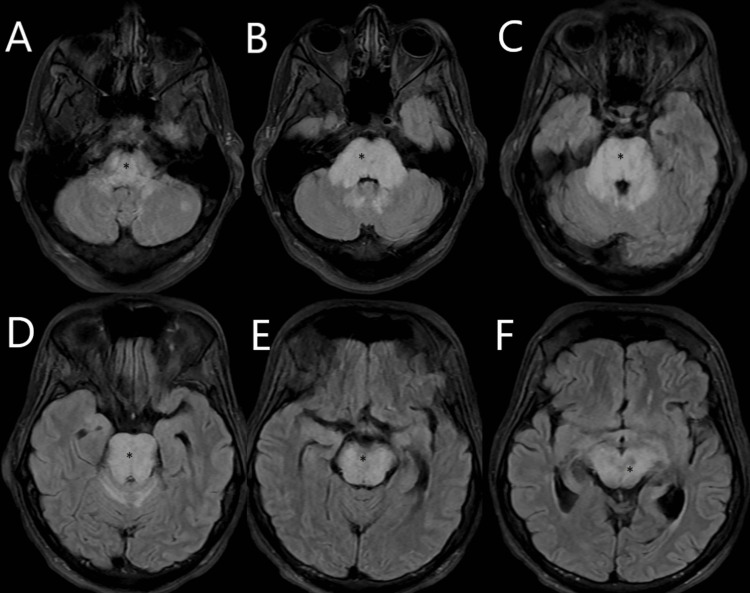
MRI brain (T2-FLAIR) with hyperintensity in the brainstem demonstrating hyperintensity diffusely involving the brainstem structures (asterisk). * hyperintensity representing edema
